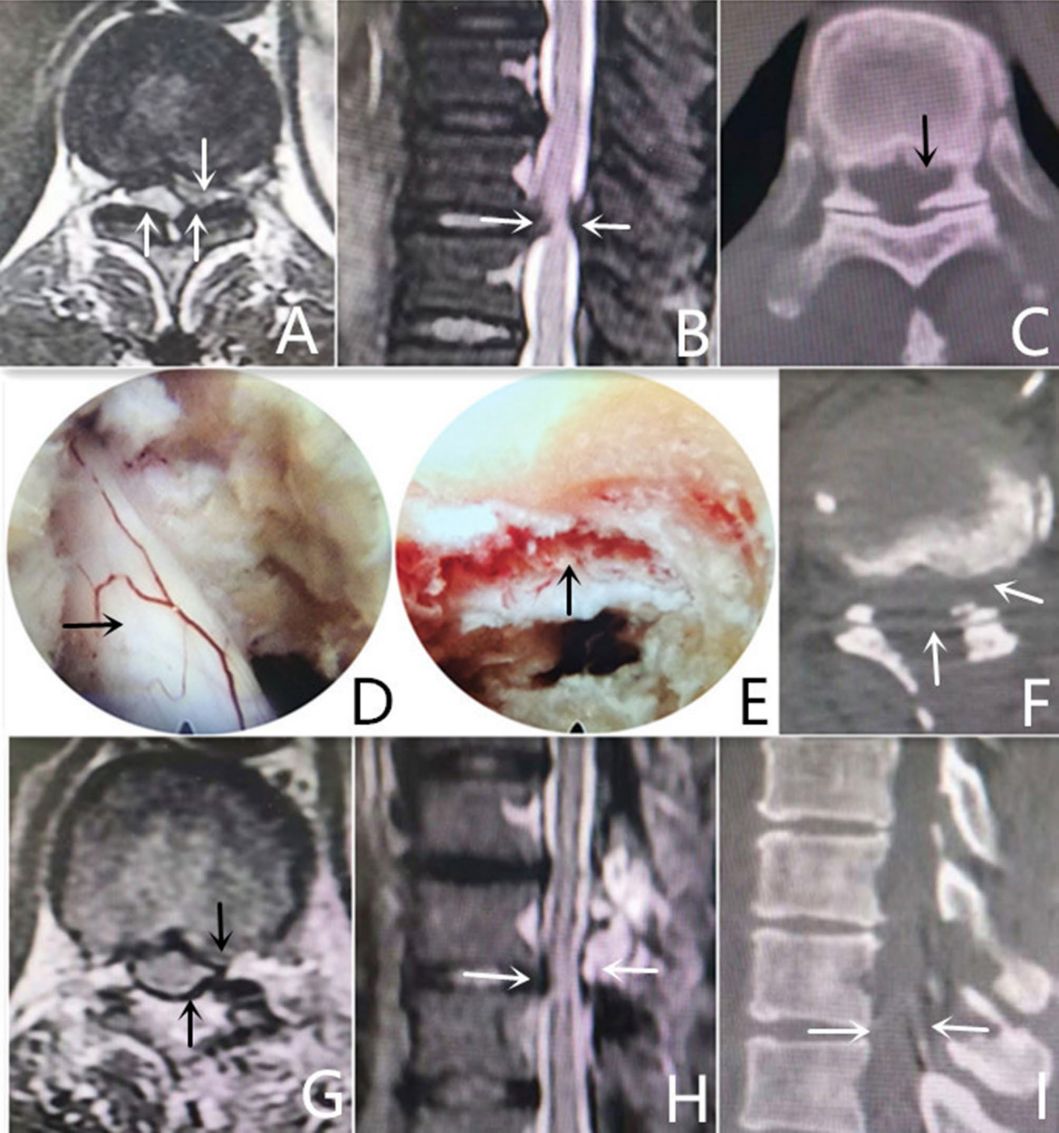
Typical cases. (A–C) Preoperative CT and MRI examinations showed T_10/11_ disk herniation on the left side with ossification of the ligamentum flavum and thoracic spinal cord degeneration in the corresponding segment. (D) Dura mater exposure after decompression via the posterior approach. (E) Dura mater margin exposure under decompression via the posterolateral approach. (F–I) Postoperative CT and MR showed that the disc herniated, the ossified ligamentum flavum was removed, and there was no compression in the dura mater.
